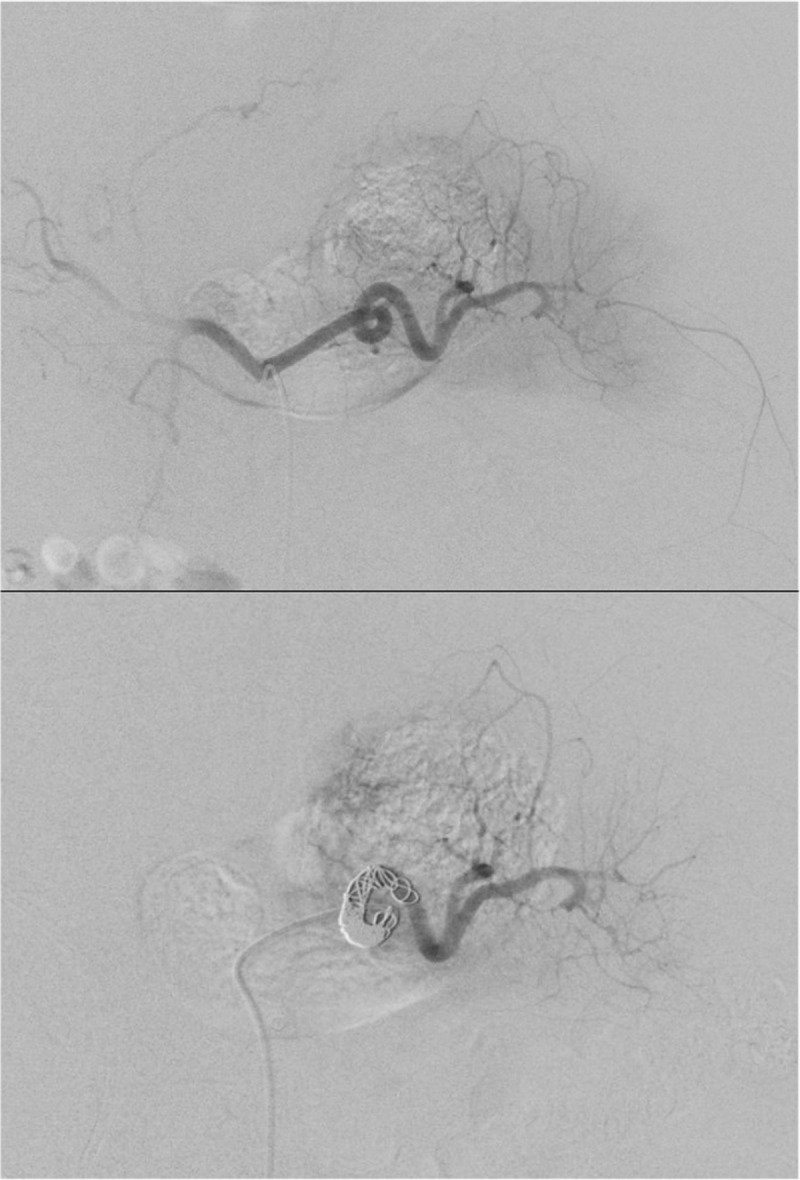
The procedure was well tolerated, without any complications. Three weeks later, repeat CT imaging showed a stable peri-splenic hematoma with multiple new splenic infarcts post embolization.
